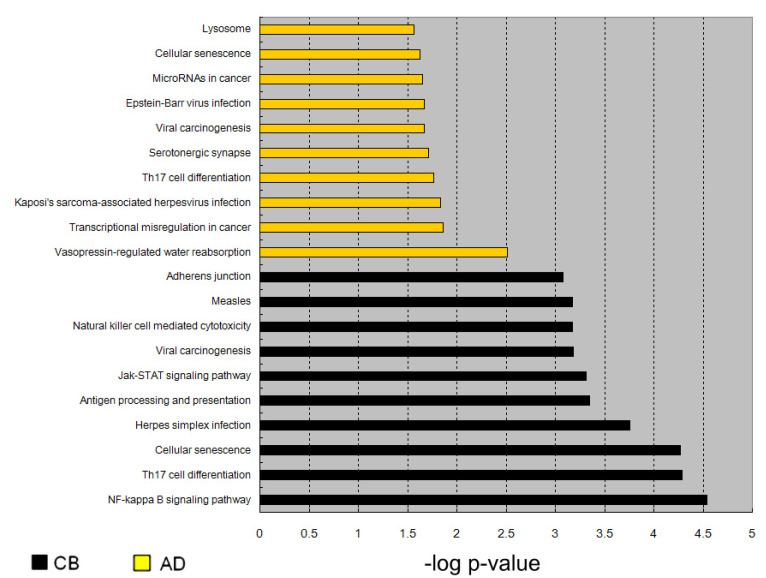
Comparisons of enriched pathways between cord blood (CB) and adult blood (AD). We identified significantly hypomethylated CpG dinucleotides (CT vs. CC, comparing the factor l-arginine treatment) and identified activated genes of cord blood and adult blood samples, followed by pathway analysis. We then compared the top-10 most significant pathways identified in either CB or AD samples.
